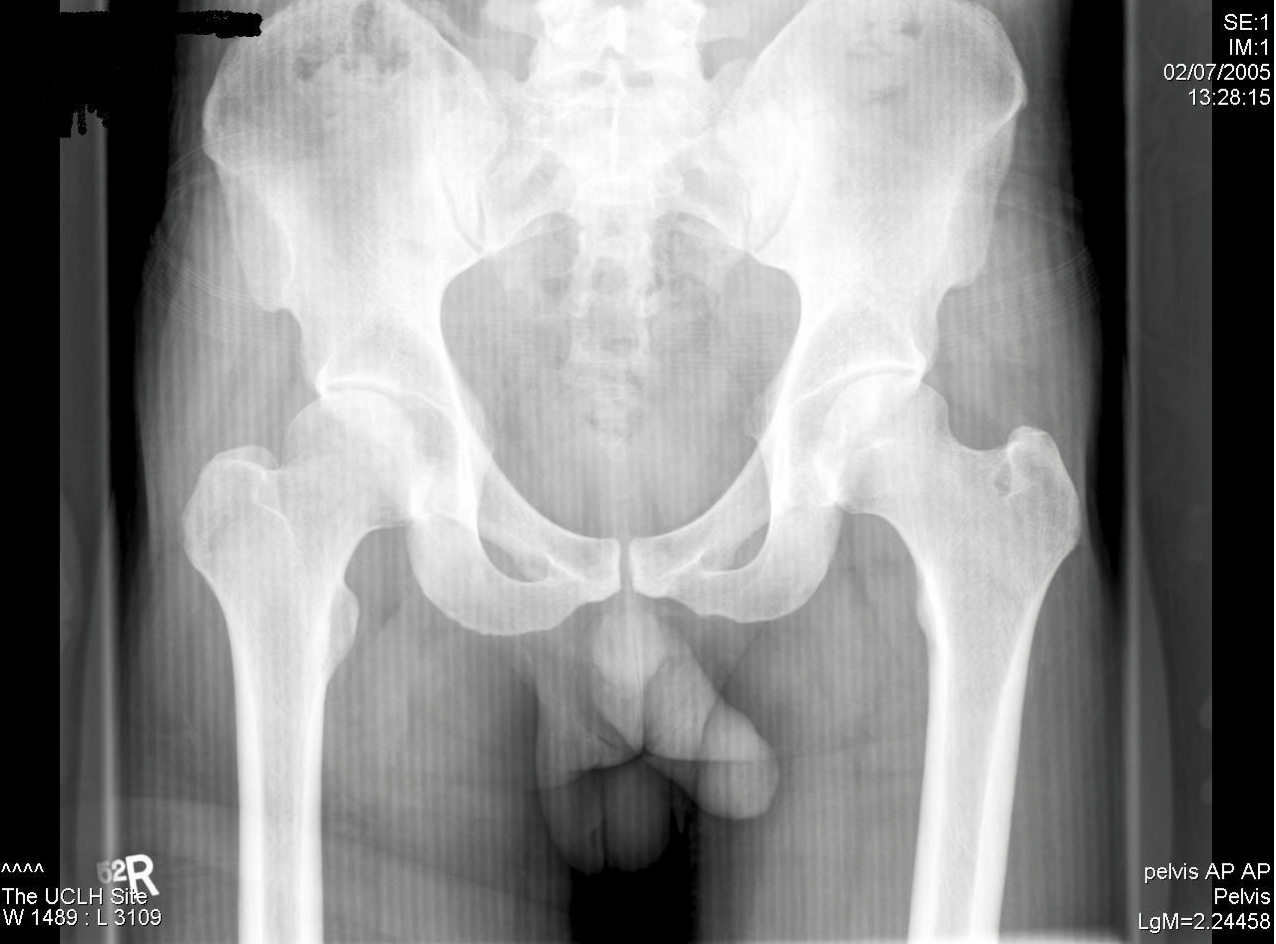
X-ray of the pelvis showing no positive findings.
